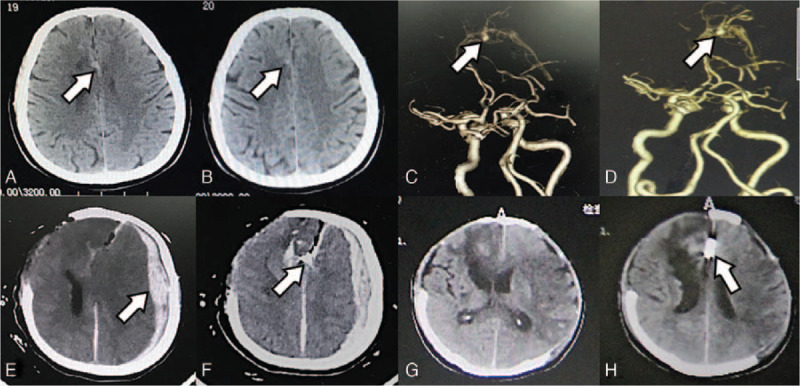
Radiological profiles of Case 2. (A–B) CT revealed hyperintensity involving the right cerebral falx. (C–D) CTA revealed an aneurysm in the distal anterior cerebral artery. (E–F) Postoperative CT revealed an acute contralateral subdural hematoma with a significant midline shift. (G) CT after the second operation and (H) repeated CT after 2 weeks revealed that the hematoma was completely evacuated. CT = computed tomography, CTA = CT angiography.
